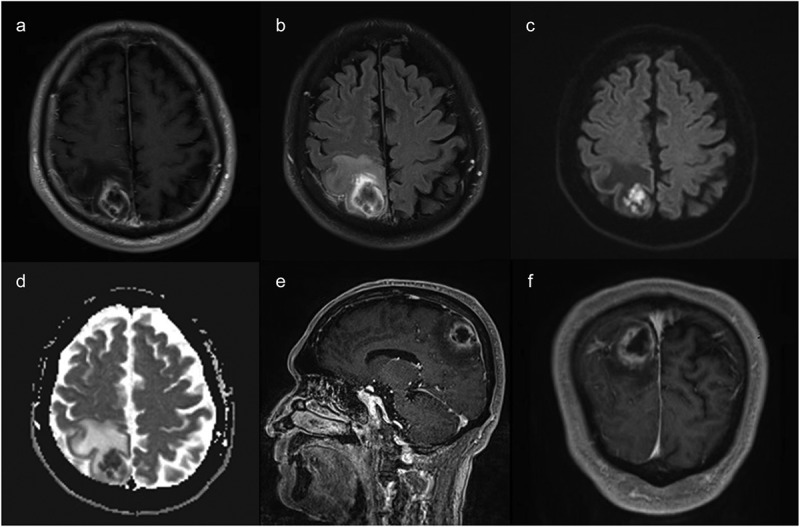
MRI of the brain after admission showed a thin rim-enhancing lesion with perilesional edema in the right parietal lobe (A.B.E.F). The lesion had internal restricted diffusion on DWI and ADC (C.D).
